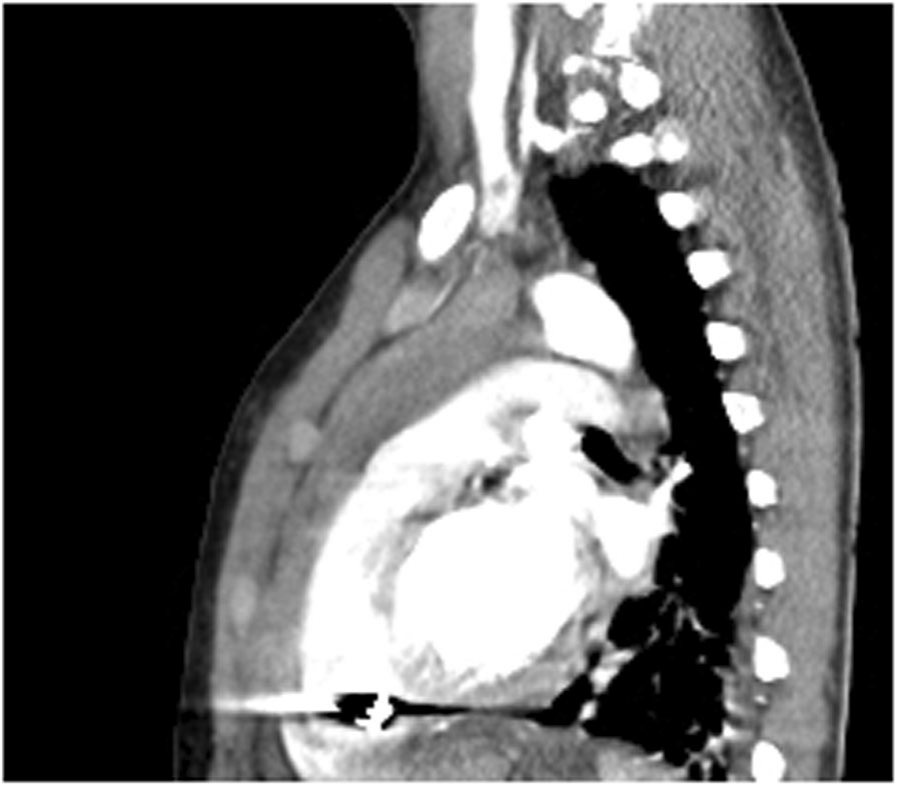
Chest CT scan with contrast, sagittal view sowing the radio-opaque pellet in the right ventricular apex with shadow artefact
